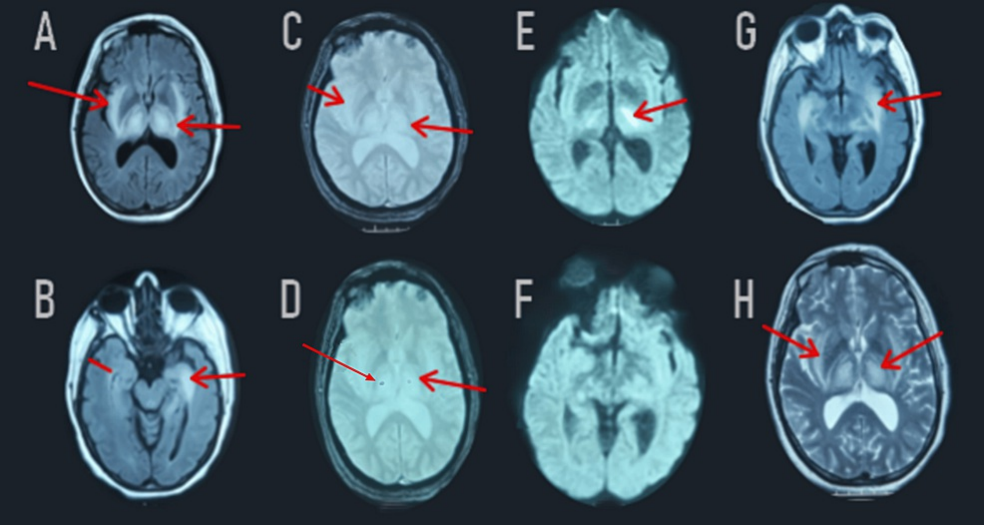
Follow-up MRI revealed significant regression of the FLAIR-weighted hypersignal in the two thalami, the cerebral peduncles, the bulb, the hippocampus, and the right internal temporal areas (A, B, G). Diffusion-weighted imaging (E, F) showed a decrease in the apparent diffusion coefficient on the periphery (red arrow). Post-gadolinium images demonstrated contrast enhancement in the two thalami (not shown). T2*-weighted images (C, D) showed a stable appearance of the bilateral thalamic hemorrhagic lesions (red arrow). FLAIR: Fluid-Attenuated Inversion Recovery; T2: T2-weighted imaging; DWI: Diffusion Weighted Imaging; ADC: Apparent Diffusion Coefficient.
